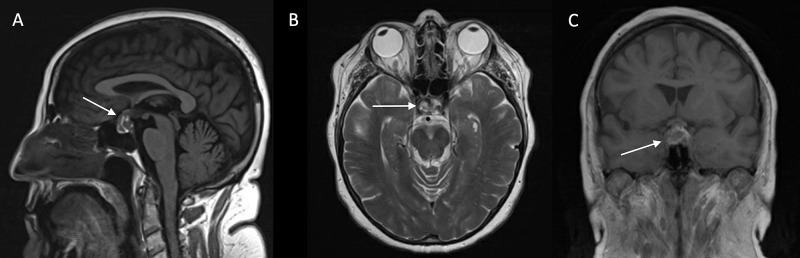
Initial MRI results Sagittal T1-weighted MRI (A) and axial T2-weighted MRI (B) images of the brain show high signal intensity suggestive of recent hemorrhage. Coronal gadolinium-enhanced T1-weighted MRI (C) revealing a 14 mm heterogeneously enhancing sellar lesion with suprasellar extension and effacing the optic chiasm. T1, longitudinal relaxation time; T2, transverse relaxation time; MRI, magnetic resonance imaging
